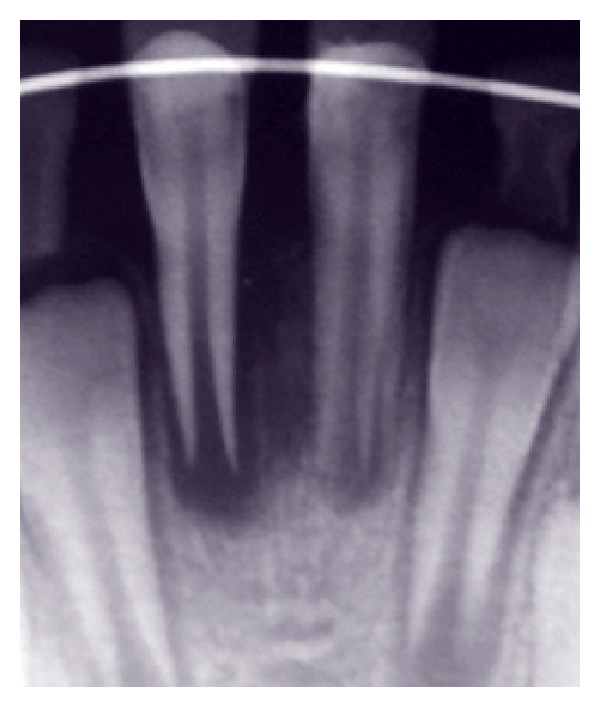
Two weeks after replantation, periapical radiolucency around the replanted tooth has increased considerably.
